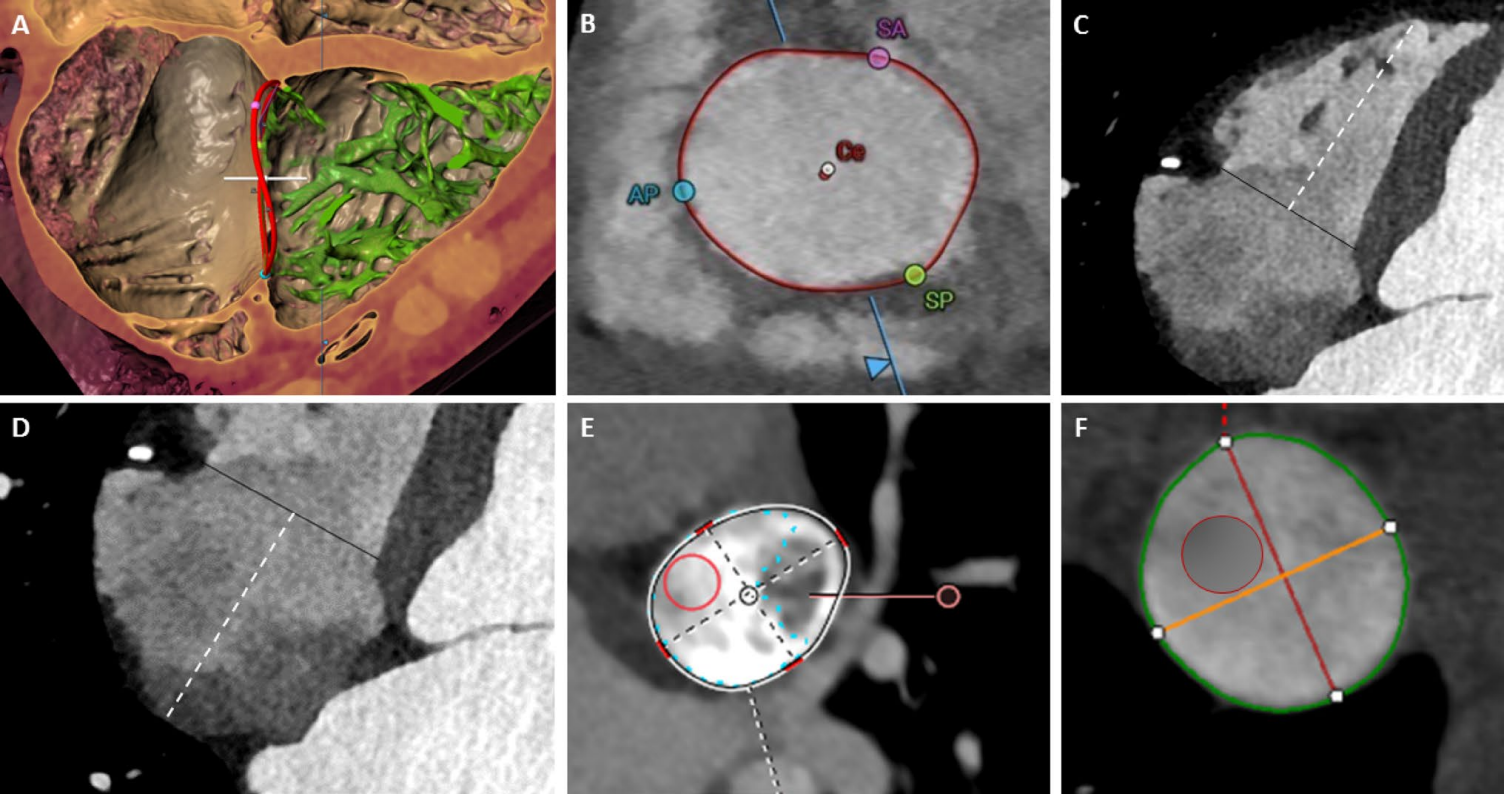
Computed tomography assessment of the tricuspid valve and right heart anatomy. After alignment of the multiplanar reformatting planes along the TV annulus and the RV apex in the orthogonal axial and coronal views, a reconstruction of the annulus (A) and planar cross-sectional area (short axis) of the annulus are obtained (B), allowing measurement of the maximal/minimal diameter, perimeter and area. 4CH view (C): Black line = Tricuspid annulus; Dashed white line = RV length. 4CH view (D): Black line = Tricuspid annulus; Dashed white line = Right atrium length. Superior vena cava diameters (E). Inferior vena cava diameters (F)
